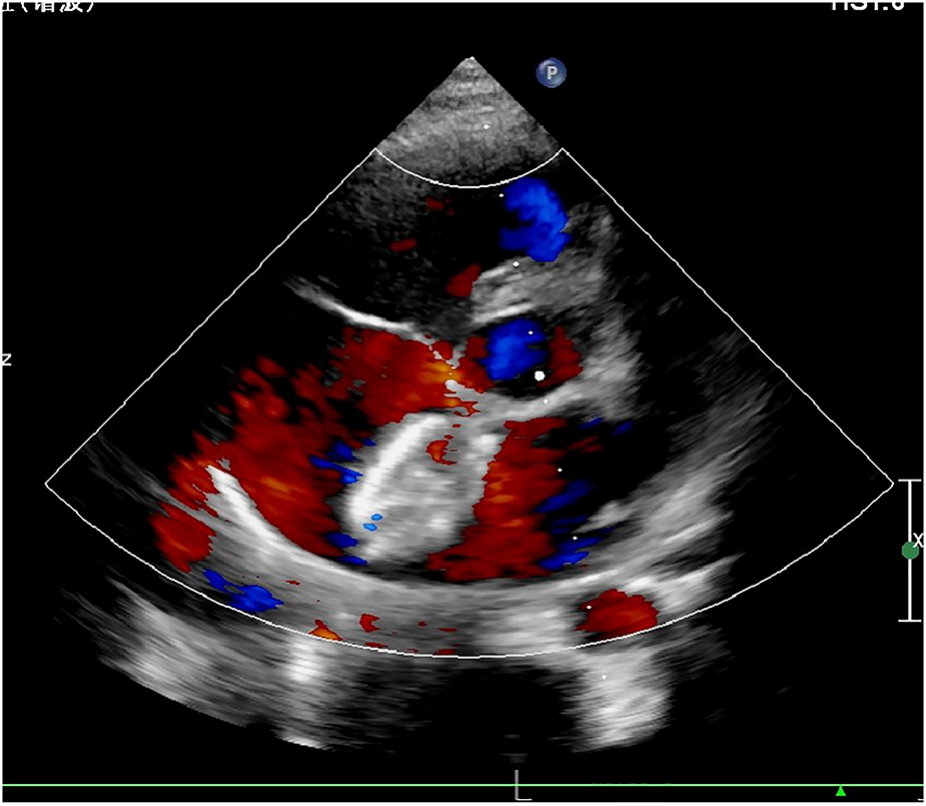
Transthoracic echocardiography at 1-month post-procedure showed that the thrombus was completely absorbed.
